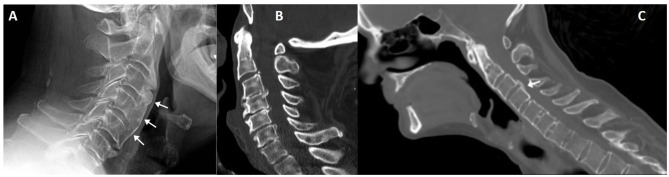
Lateral cervical radiography of a patient with cervical undulating anterior longitudinal ligament ossification (arrows), compatible with DISH (A). Another patient (B) with cervical DISH and ossification of the posterior longitudinal ligament; differential diagnosis with posterior syndesmophytes is not always straightforward, as seen in a CT sagittal reconstruction of a patient with ankylosing spondylitis [(C), arrow].
